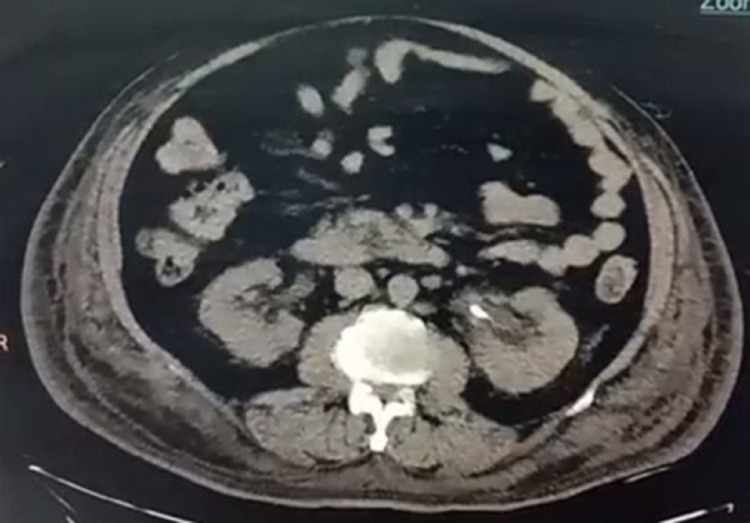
NCCT abdomen showing bilateral perirenal and periureteric fat stranding with left double J stent in situ. NCCT: Non-contrast computed tomography.
